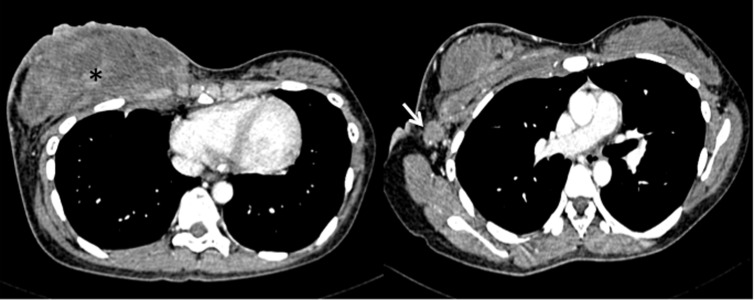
Axial enhanced CT image of the chest showing a large right breast mass (asterisks) that has infiltrated the underlying medial chest wall and a large right axillary lymph node (arrow).
